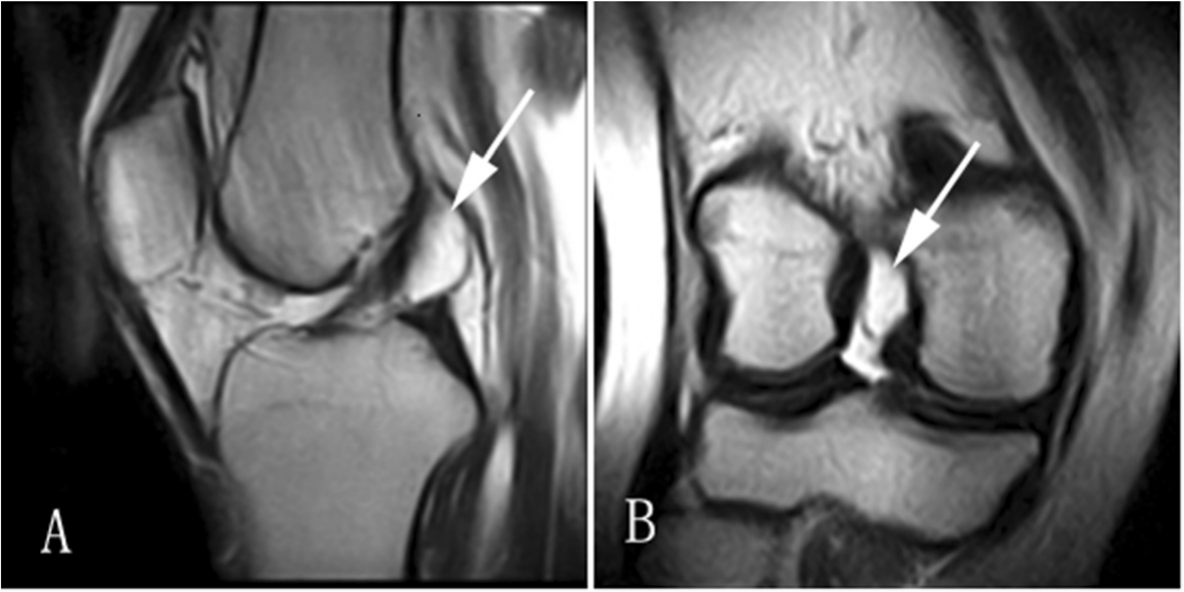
Cyst of the ACL on T2-weighted image. (A) The ACL was twisted, due to presence of the cyst in the sagittal plane. (B) The cysts of the ACL in the coronal plane.
